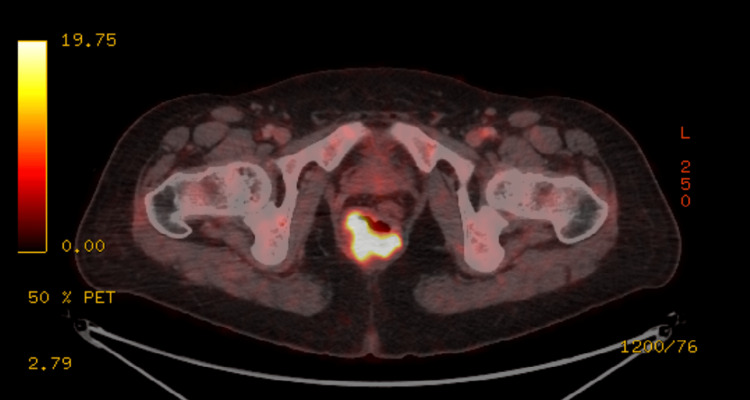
PET-CT demonstrating pathological signal on the right side of the anus and the perianal area PET-CT, positron emission tomography-computed tomography
